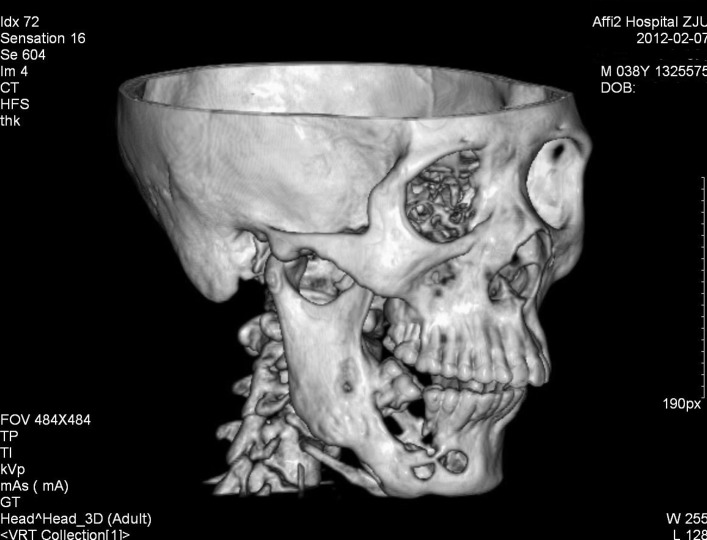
Computed tomography (CT) scan confirming the continuity of the mandible eight months later.
